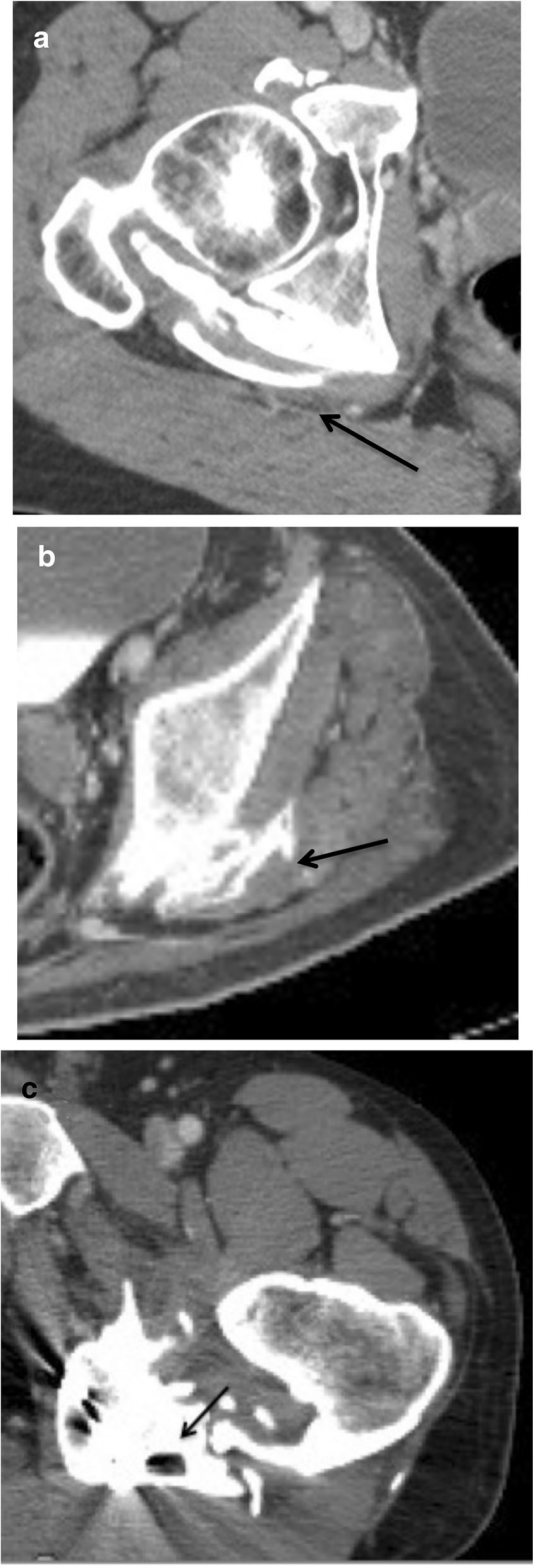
Axial images of a biphasic enhanced CT in three different patients with posterior osteomas with various types of relationships with sciatic nerves. a slight contact and compression of the right sciatic nerve by the osteoma (*arrow*). b the osteoma forms a groove around the sciatic nerve, surrounding it less than 180° (*arrow*). c the osteoma forms a complete bony tunnel around the sciatic nerve (19 year-old male patient) (*arrow*)
